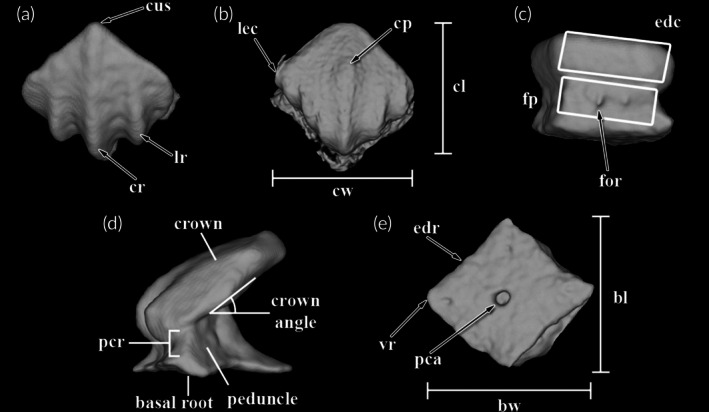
Terminology (see Terminology section in Results). (a, b) Dorsal view of denticles. (c) Frontal view of denticle. (d) Lateral view of denticle. (e) Ventral view of denticles. Bl, base length; bw, base width; cl, crown length; cw, crown width; cp, central peak; cr, central ridge; cus, cusp; edc, edge of the crown; edr, edge of the basal root; for, foramen; fp, face of the peduncle; lr, lateral ridge; pca, pulp cavity; pce, peduncle crest; vc, vertex of the crown; vr, vertex of the peduncle. In (a, b, e, f) anterior to bottom; In (c) anterior to front; In (d) anterior to left (micro CT‐scan).
